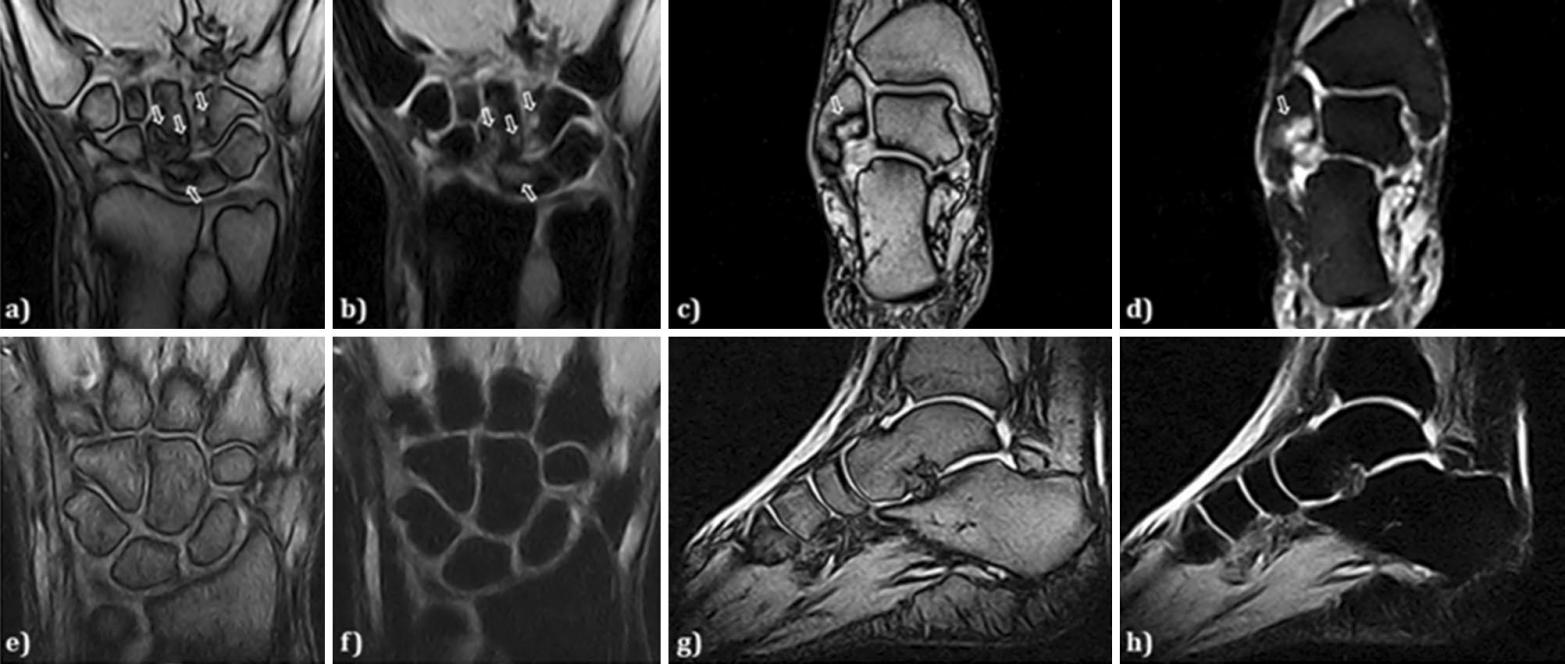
Synovitis, bone oedema, and bone erosions, as common MRI findings on wrists and ankles in AOSD. In panels (a) and (b), T1-weighted and T2-STIR images of coronal sections on wrist are shown, respectively, detailing a mild to moderate proliferative synovitis, as thickening of the synovial membrane, with low to intermediate signal intensity on T1-weighted images and intermediate to high signal intensity on T2-STIR images (arrows). Bone oedema and bone erosions are also shown, which overlapped the locations (arrows). In panel (c) and (d), T1-weighted and T2-STIR images of coronal sections on ankle are shown, respectively, detailing a mild to moderate proliferative synovitis, with low to intermediate signal intensity on T1-weighted images and intermediate to high signal intensity on T2-STIR images (arrows). Bone oedema and bone erosion are also shown, which overlapped the locations (arrows). In panels (e) and (f) T1-weighted and T2-fat saturated images of coronal sections on wrist are shown, respectively, detailing no pathologic findings. In panels (c) and (d), T1-weighted and T2-STIR images of sagittal sections on ankle are shown, respectively, detailing no pathologic findings.
